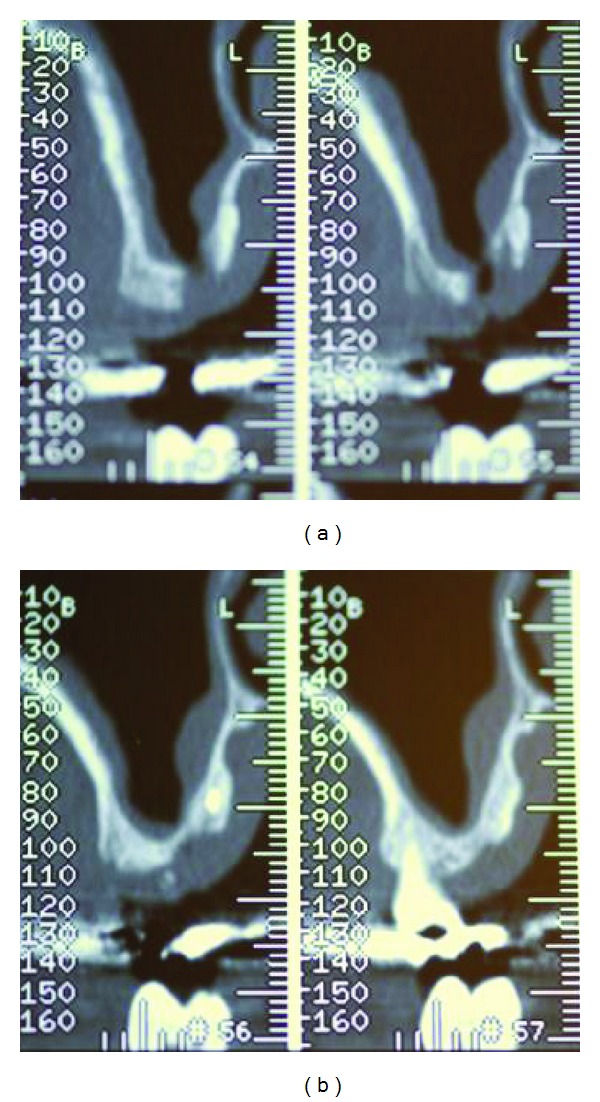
((a), (b)) Radiographic exams (paraxial images) also revealed not only the residual root apex in the palatal side, but also insufficient bone volume.
